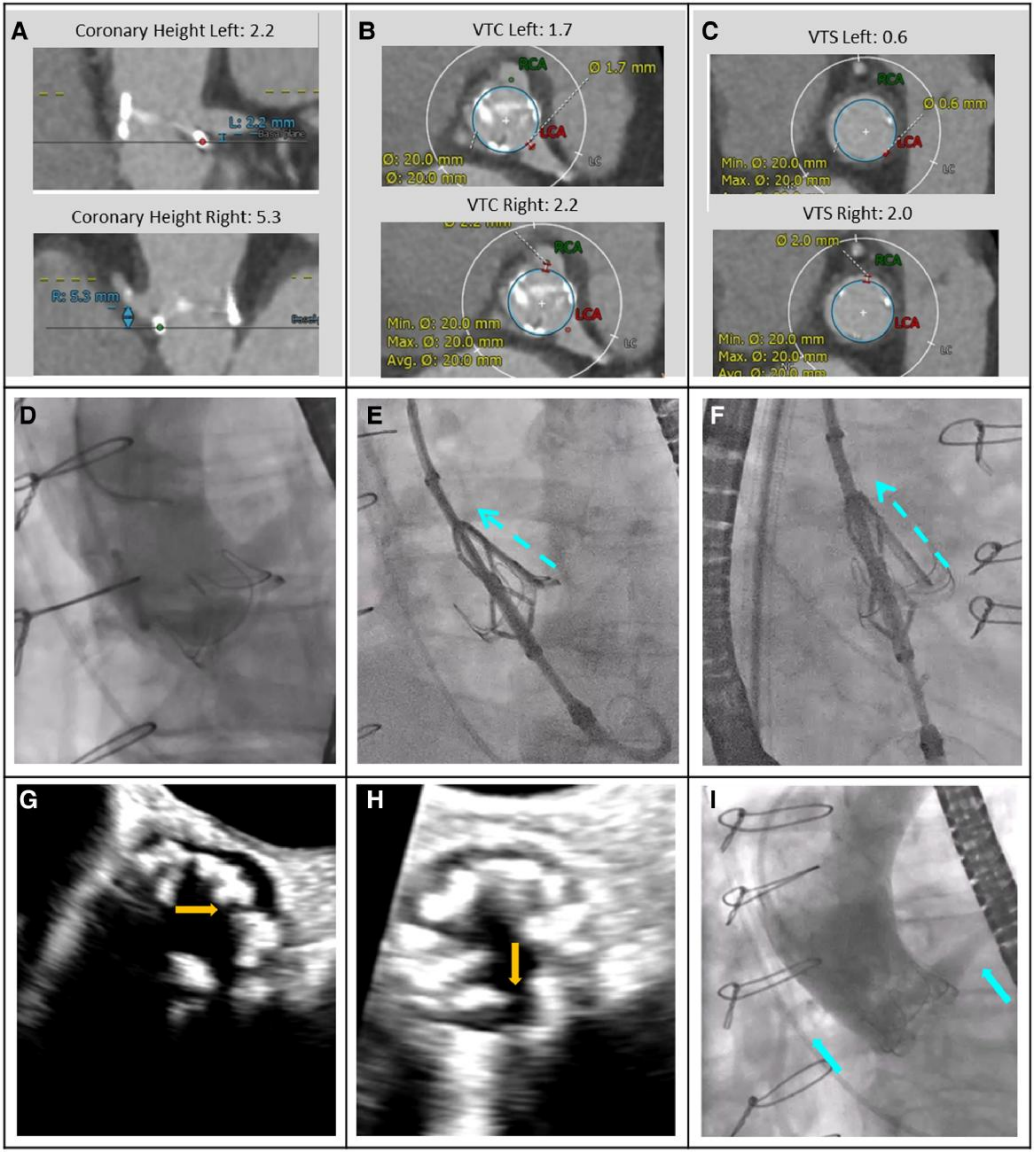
A ShortCut procedure in a patient with a failed bioprosthetic surgical valve. A 76-year-old female with a failed Magna Ease 21 mm surgical bioprosthetic aortic valve (Edwards Lifesciences) at high risk for double coronary obstruction that was treated with ShortCut and Evolut R 23 mm (Medtronic). (*A–C*) Computed tomography measurements showing predicted risk of coronary obstruction. (*D*) Baseline aortogram demonstrating risk for coronary obstruction (side view of left and right coronary cusps). (*E* and *F*) The ShortCut is positioned. Activated splitting element punctures the leaflet from the ventricular side just above the annulus. The leaflet is split by gently retracting the catheter while feeding the guidewire. (*G* and *H*) Echocardiography verifies left and right leaflet split (annotated arrows). (*I*) Post-TAVI implantation and ring fracture with a 23 mm True balloon (Bard) demonstrating normal coronary flow (annotated arrows). Echocardiography post-TAVI (not shown) showed aortic valve gradients of 15/6 mmHg. The patient had an uneventful hospital stay
